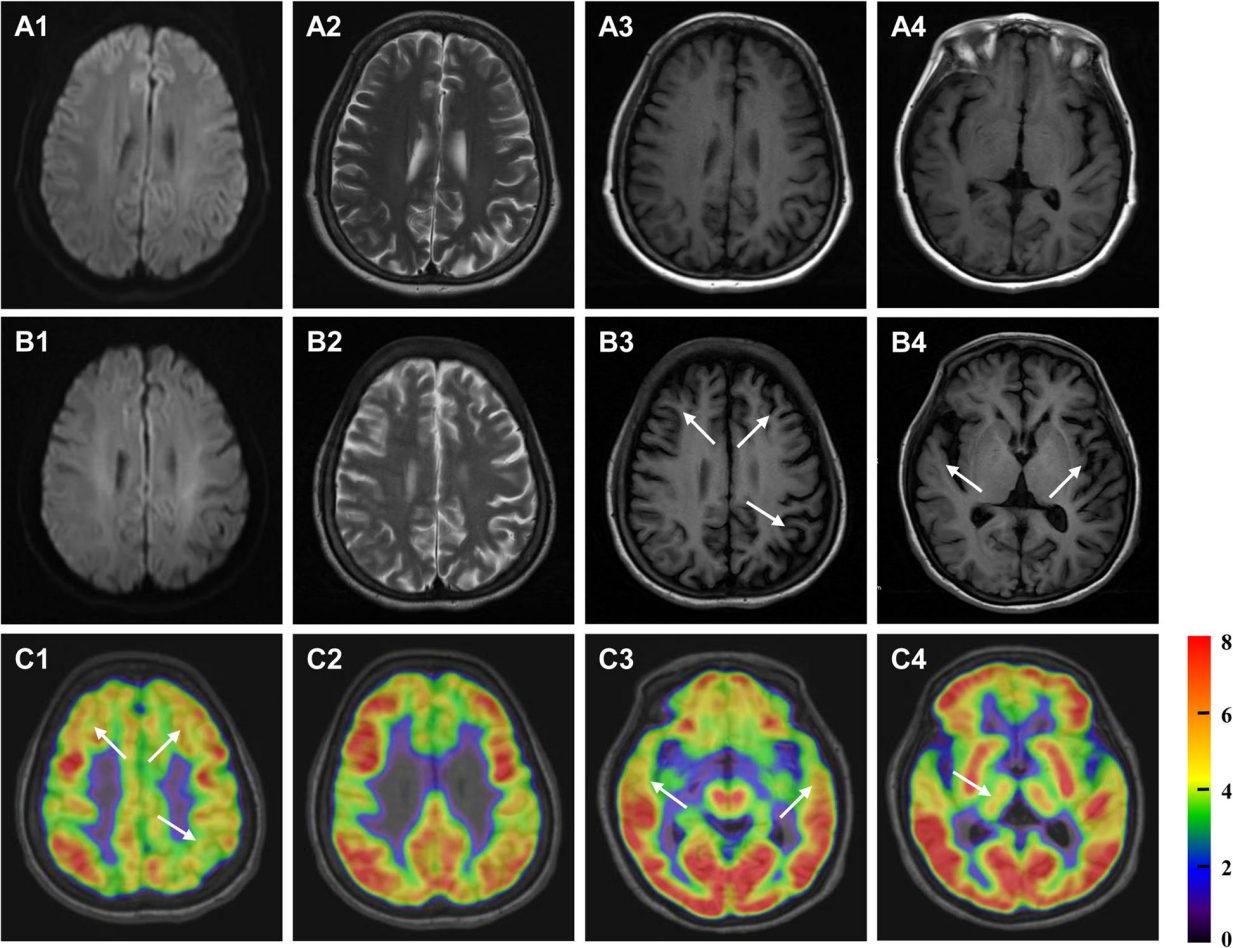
Brain MRI and ^18^F-FDG PET/MR examination. A1-A4 First brain MRI at onset showed no apparent cerebral atrophy and abnormal signals on DWI and T2WI. B1-B4 Second brain MRI five years later after onset, revealed atrophy of left parietal lobe, bilateral frontal lobes, and bilateral temporal lobes, but still without high-intensity lesions on DWI and T2WI. C1-C4
